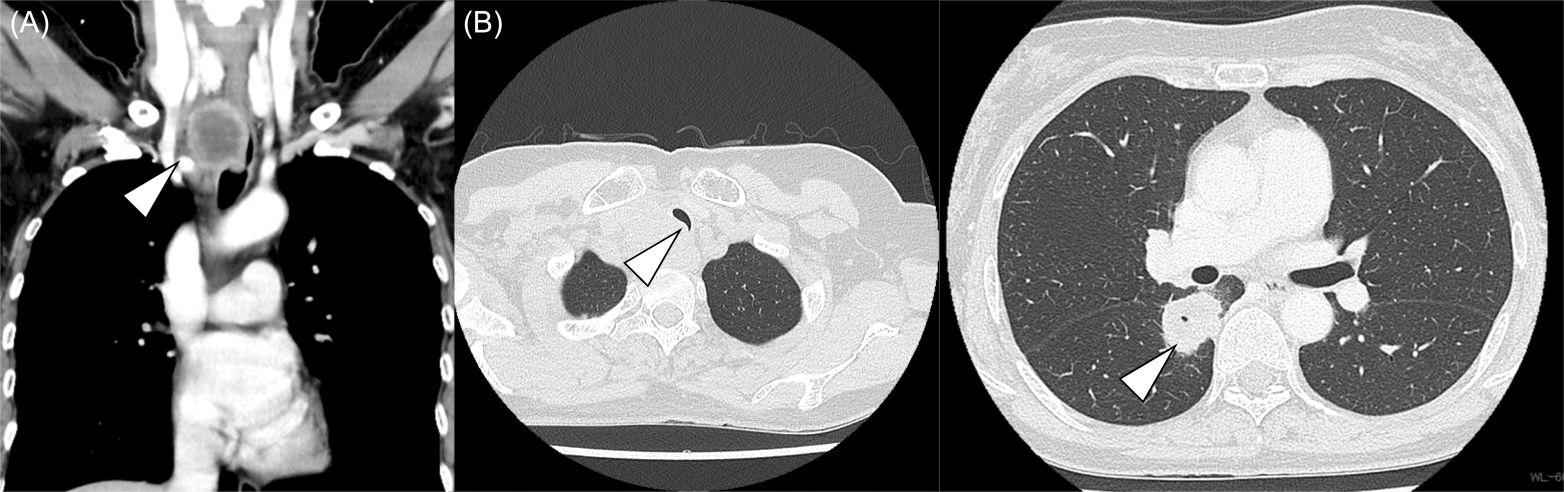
Contrast‐enhanced computed tomography (CE‐CT) on admission. (A) CE‐CT shows enlarged lymph nodes and stenosis of the upper‐to‐mid trachea (white arrow). (B) Chest CT shows a 3‐cm mass in the S6 segment of the right lower lobe.
